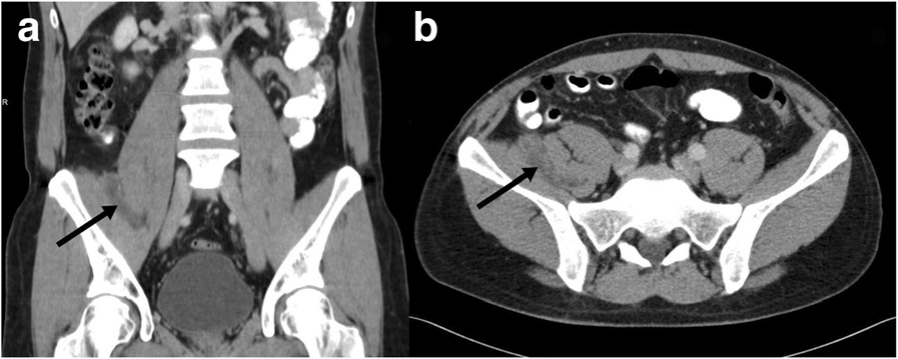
48-year-old man with right-sided retroperitoneal hibernoma. Tumour between the psoas and iliac muscles (arrows). Contrast enhanced CT shows minimal heterogeneous enhancement of the tumour. a coronal reconstruction, b axial slice.
